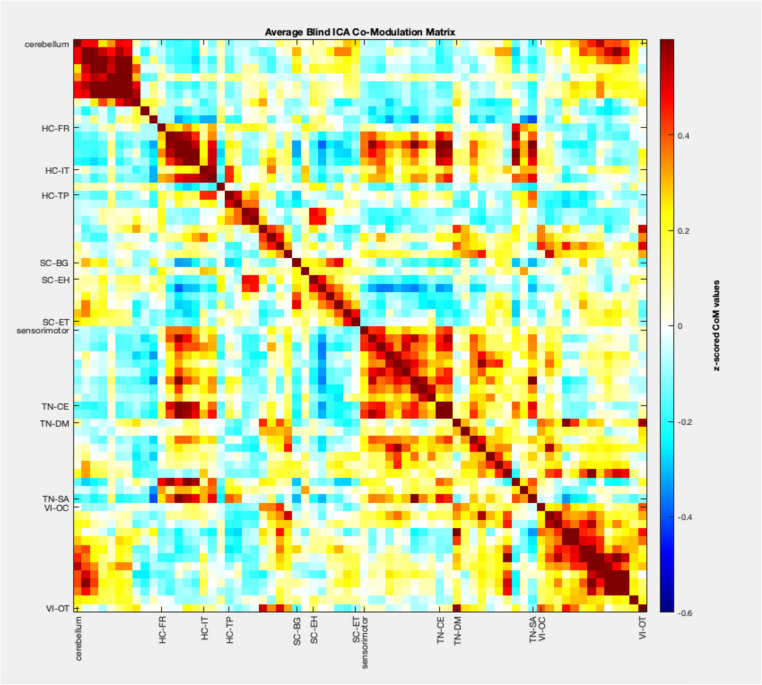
Average CoM matrix from the first run of blind ICA utilized to create the NeuroMark SPECT template. Here, the 68 components are organized by the respective NeuroMark 2.2 domains and subdomains listed as the following: cerebellar (CB), higher cognition- frontal (HC-FR), higher cognition – insular-temporal (HC-IT), higher cognition-temporoparietal subdomain (HC-TP), subcortical-basal ganglia (SC-BG), subcortical – extended hippocampal (SC-EH), subcortical – extended thalamic (SC-ET), sensorimotor (SM), triple network – central executive (TN-CE), triple network – default mode (TN-DM), triple network-salience (TN-SA), visual-occipital (VI-OC), and visual-occipitotemporal (VI-OT) subdomains. Results show highly modular organization, with both short (within domain) and long (between domain) range covariation
